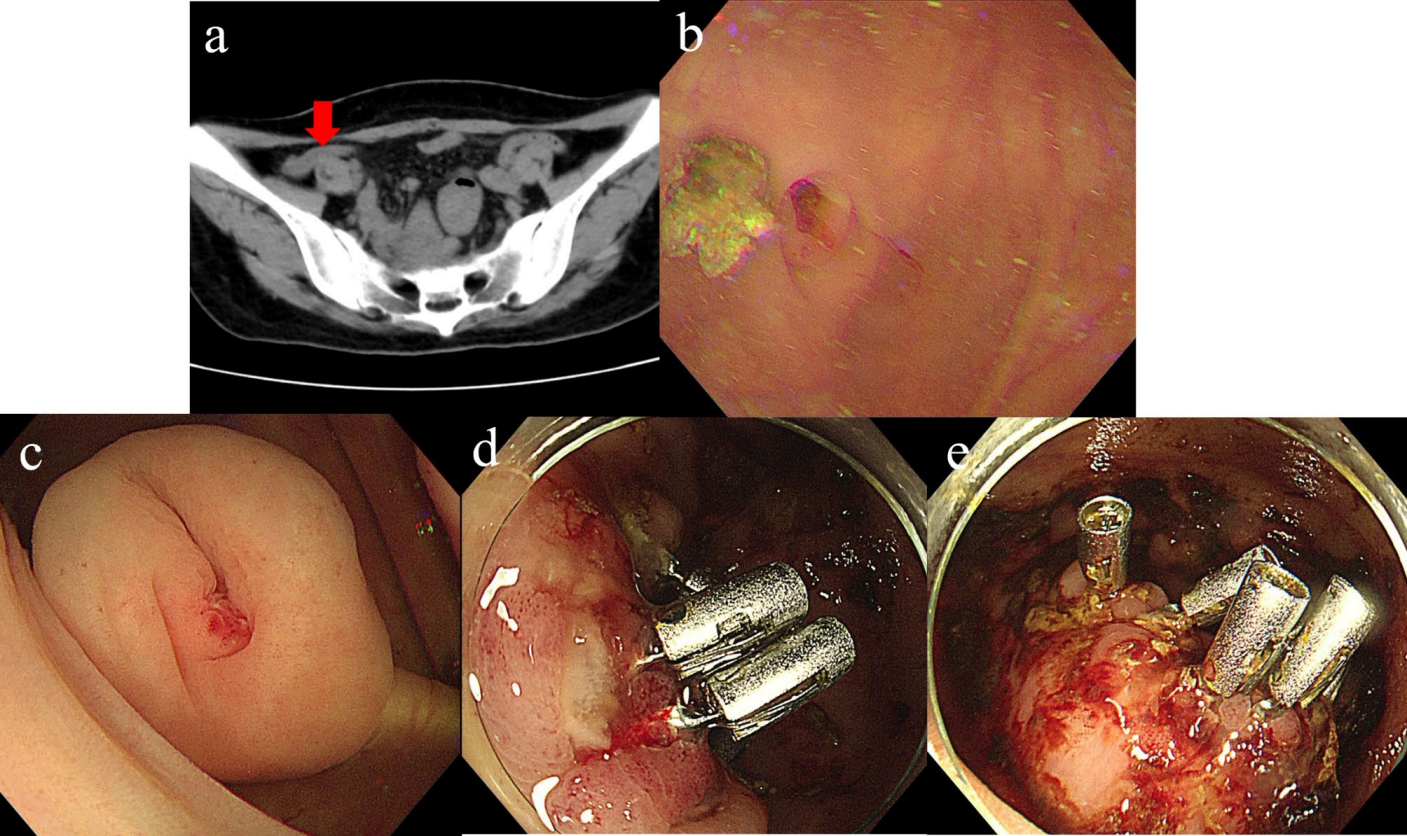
Appendicitis associated bleeding: (a) A long thickening appendix (red arrow) was shown in the contrast-enhanced CT image. (b) After removing the fecaloma away, fresh blood flowed from the orifice of appendix. (c) Ulcer with visible vascular stumps at the edge of the remaining appendix was seen. (d-e) Multiple hemostatic clips with 0.2-0.4 cm oozing ulcers were seen at the basement
